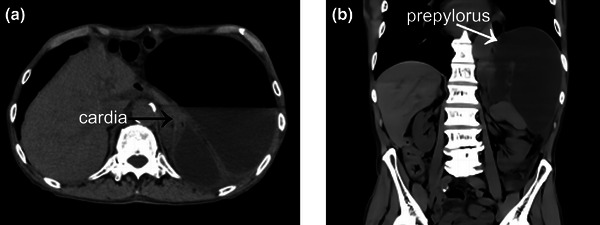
Plane abdominal computed tomography findings: (a) Horizontal section: The black arrow indicates the cardia. (b) Coronal section: The prepyloric region is inverted and displaced to the left of the aorta
